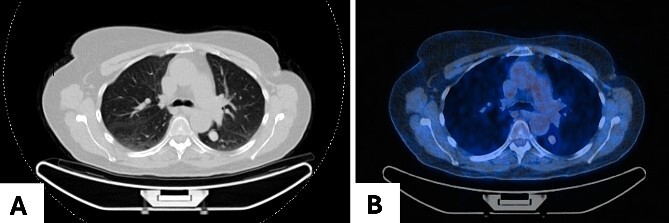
Pulmonary meningioma in segment VI of the left lower lobe in axial view in (A) CT scan showing a well-circumscribed/bordered homogenous, solid, noncalcified lesion; (B) PET-CT scan showing minimal metabolic activity.
